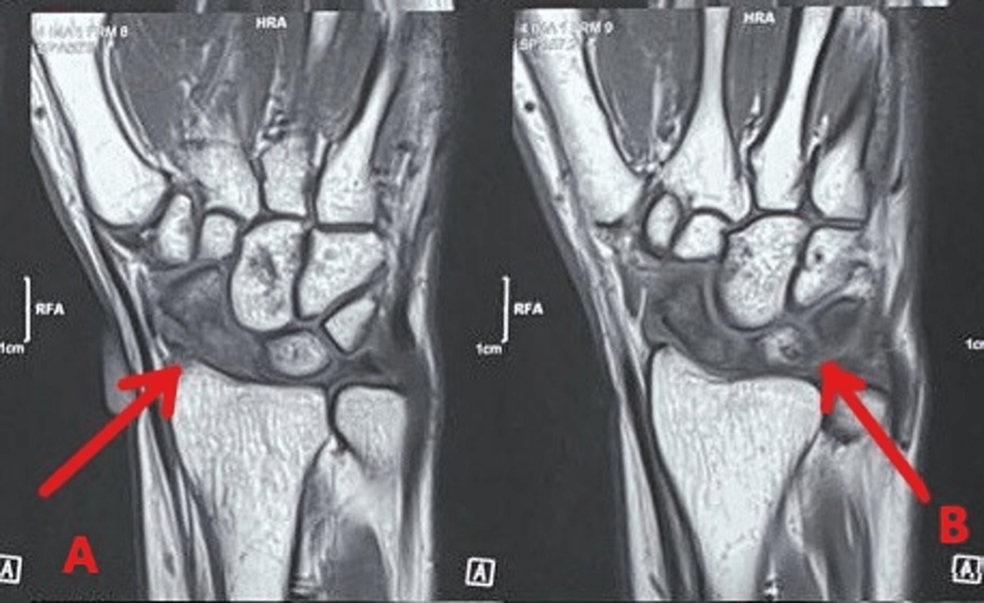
MRI showing diffuse altered marrow signal of the scaphoid. A: MRI showing altered signal in the scaphoid. B: MRI showing altered signal in lunate and triquetrum.
